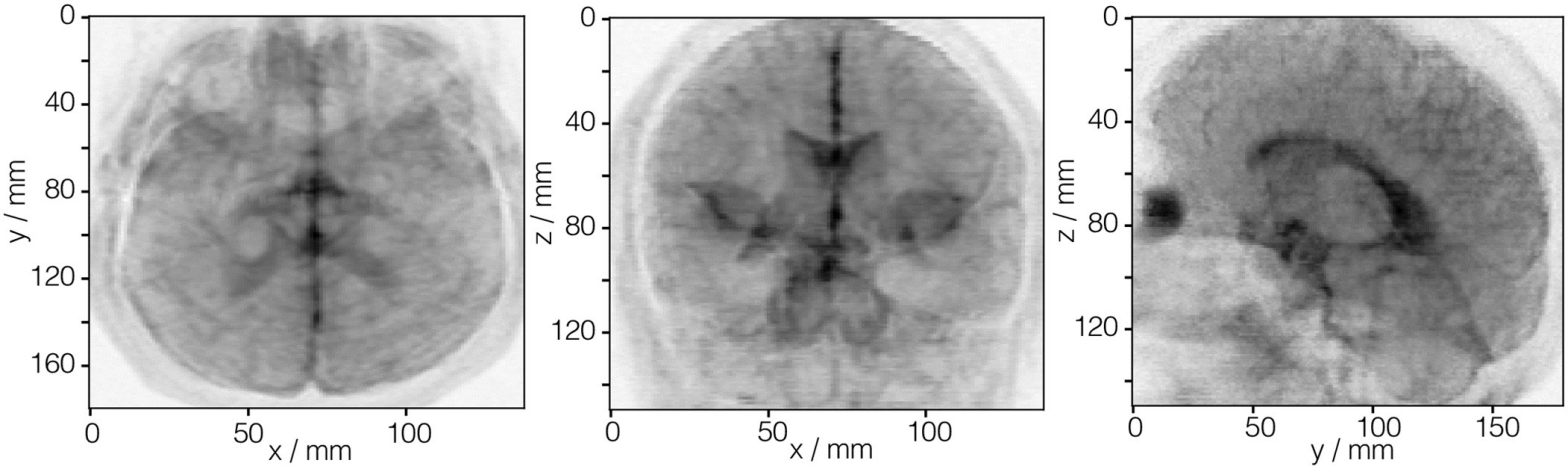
CSD model depiction of an MRI tensor dataset. The intensity plots, derived from the diffusion tensor MRI dataset in Listing 11, are the projection of the isotropic diffusion coefficients, calculated on to the three spatial dimensions. The figure was created by the authors using data from reference [17]. The diffusion tensor MRI Brain dataset [17] courtesy of Gordon Kindlmann at the Scientific Computing and Imaging Institute, University of Utah, and Andrew Alexander, W. M. Keck Laboratory for Functional Brain Imaging and Behavior, University of Wisconsin-Madison.
